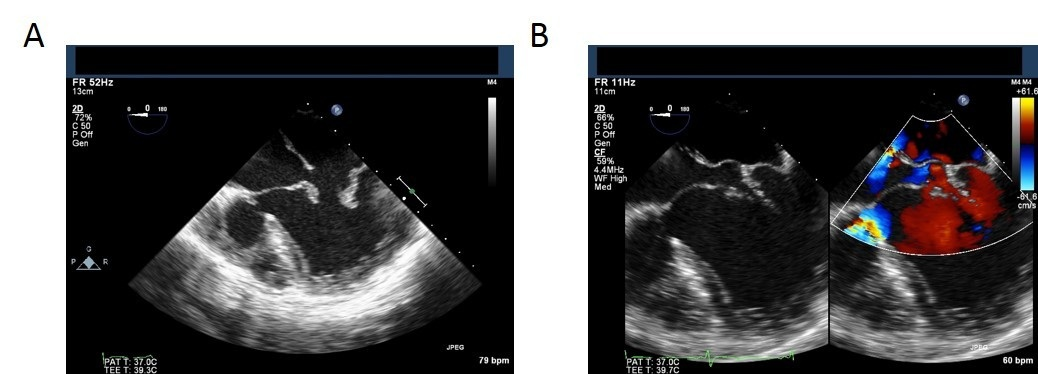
Intra-operative Transesophageal Echocardiogram Patient 2 (A) Double inlet left ventricle (B) ASD, VSD and double outlet left ventricle
